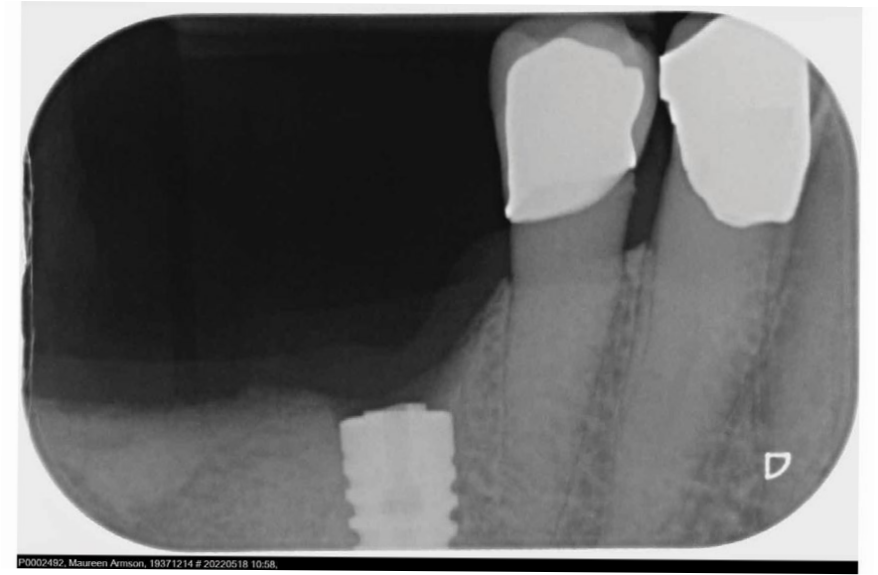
Periapical radiograph showing the apical portion of the fractured abutment still in the implant once the loose coronal portion has been removed
